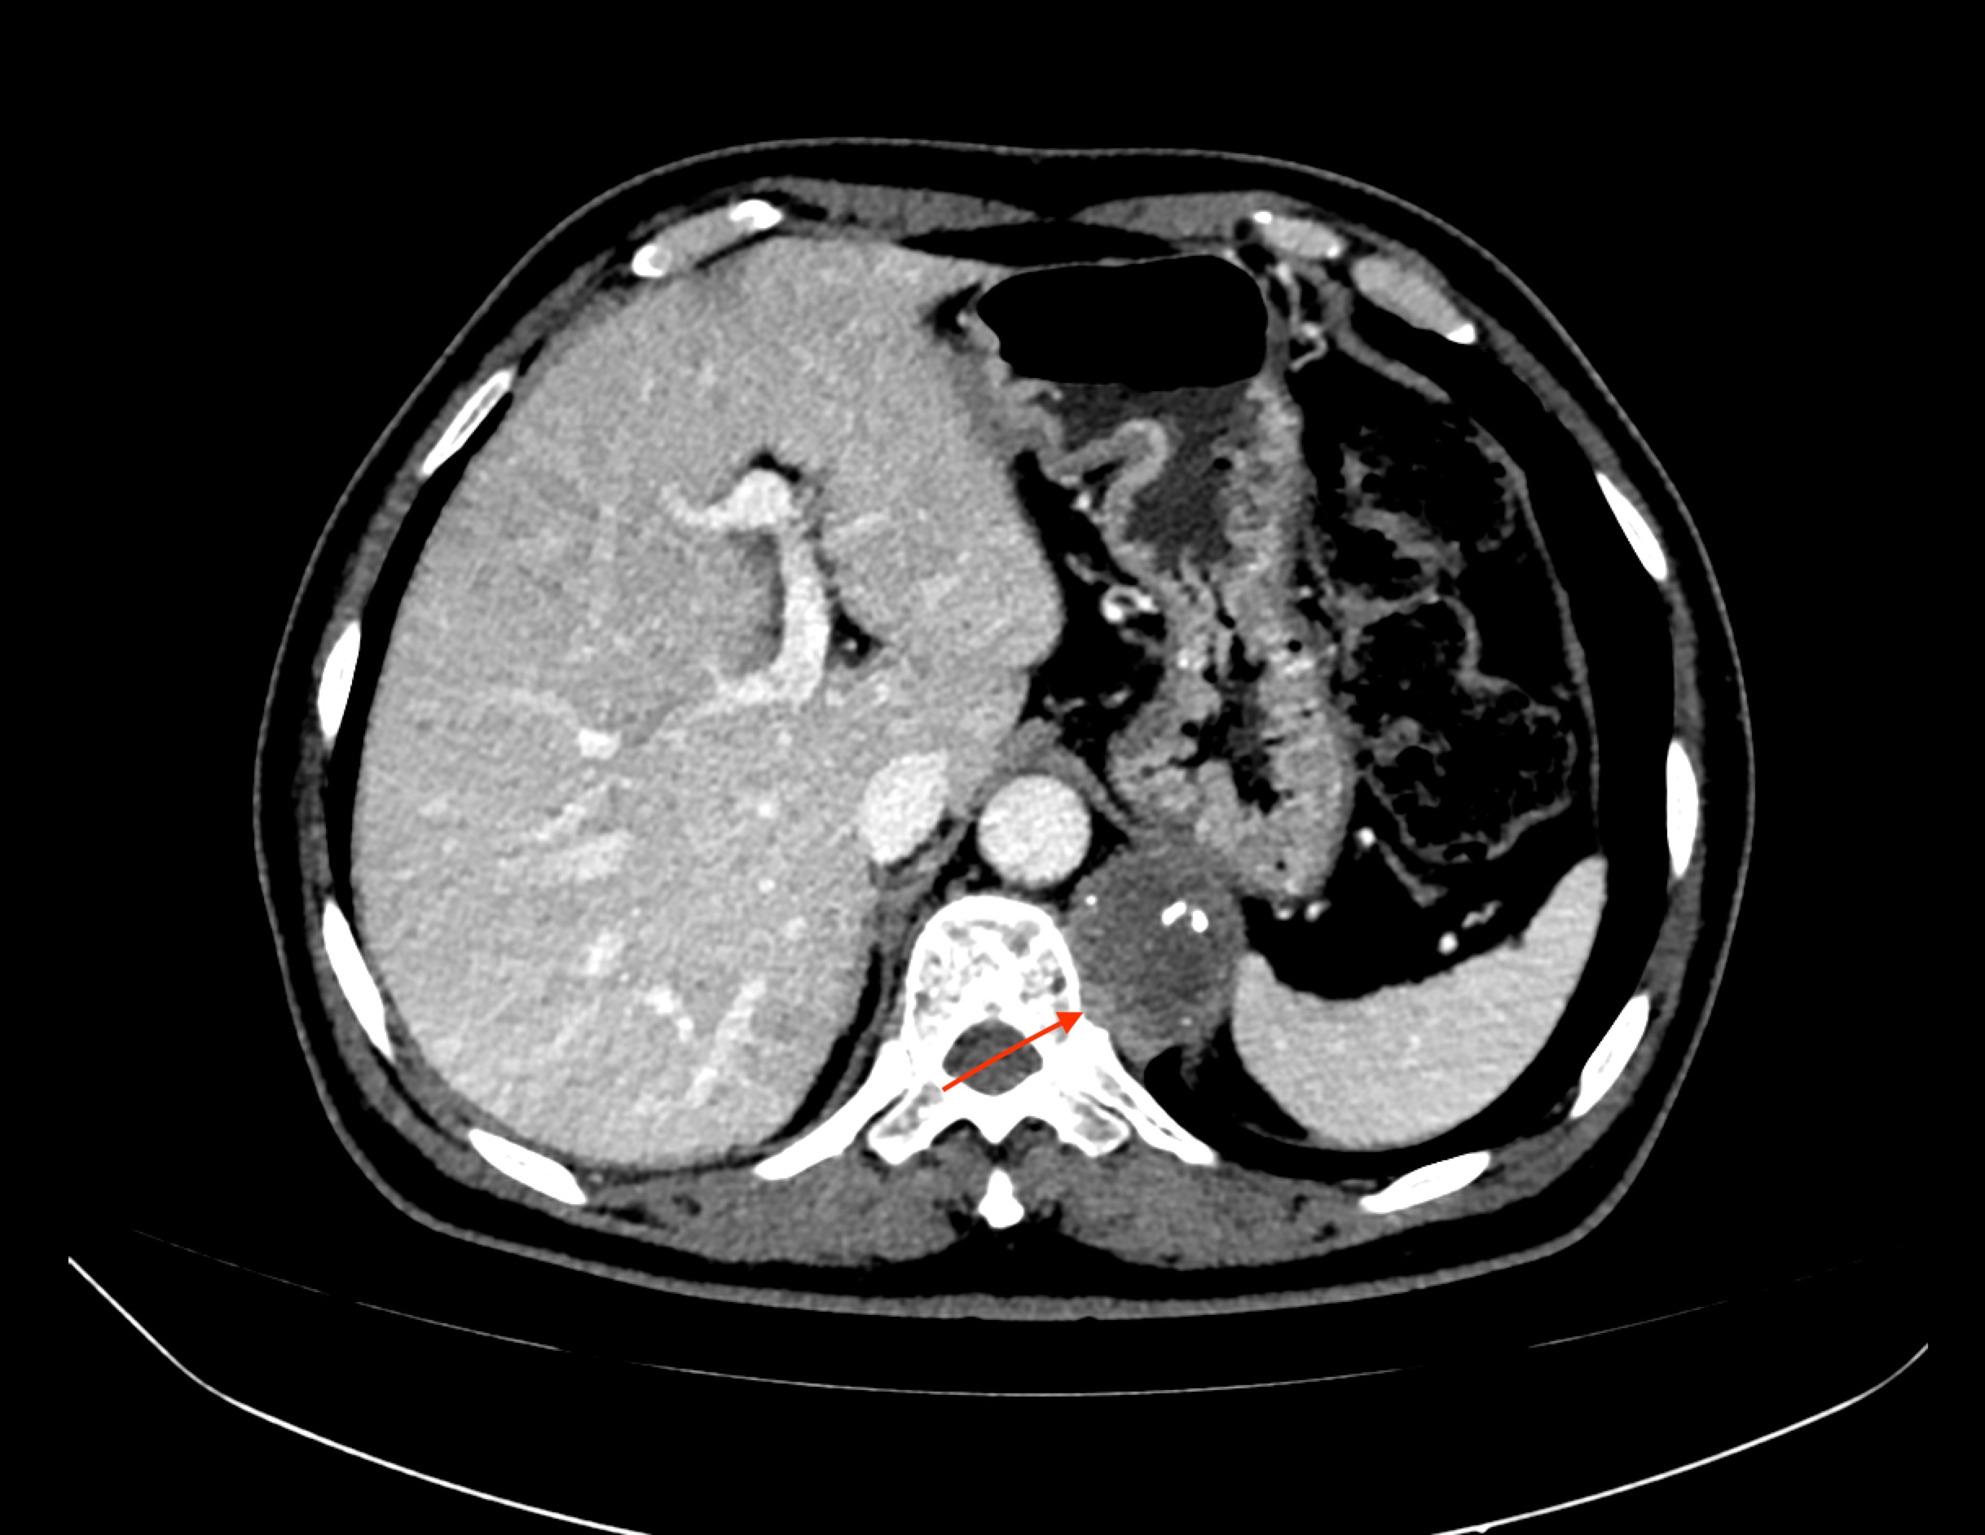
Preoperative chest computed tomography (CT). Contrast-enhanced CT imaging reveals a well-defined, heterogeneous mass measuring approximately 5.2 × 4.1 cm located in the left posterior mediastinum, adjacent to the thoracic vertebrae
